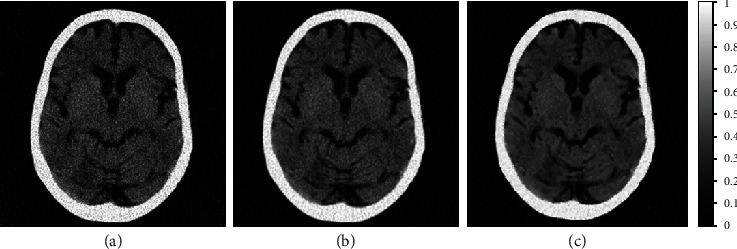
Comparison of sparse-view FBP reconstructed image with classical and proposed amoeba schemes, applied on clinically reconstructed CT image. (a) 2-degree angular sampling-based sparse-view FBP reconstruction. (b) Classical amoeba filtering-based image denoising of (a). (c) Proposed scheme with RBS amoeba denoising of (a) using Wiener pilot image.
